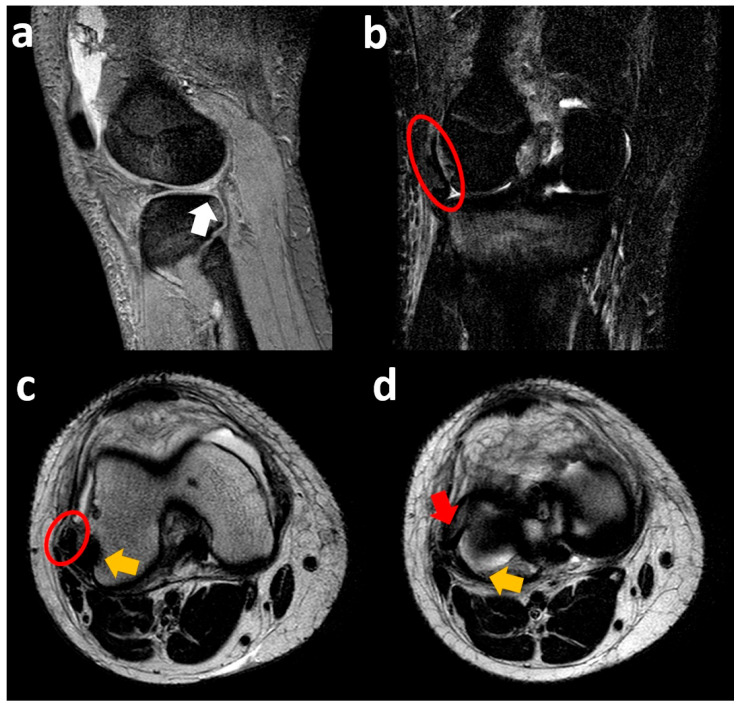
Right knee sagittal fat-suppressed T2-weighted MR image (a) shows a complete absence of the posterior horn of the lateral meniscus (white arrow). Coronal fat-suppressed T2-weighted MR image (b) shows the posterior horn meniscal flap (red circle) displaced laterally to the lateral femoral condyle. Two adjacent slices of axial T2-weighted MR images show the meniscal portion (red circle and red arrow) trapped between the popliteus tendon (yellow arrows) and the joint capsule (c,d).
